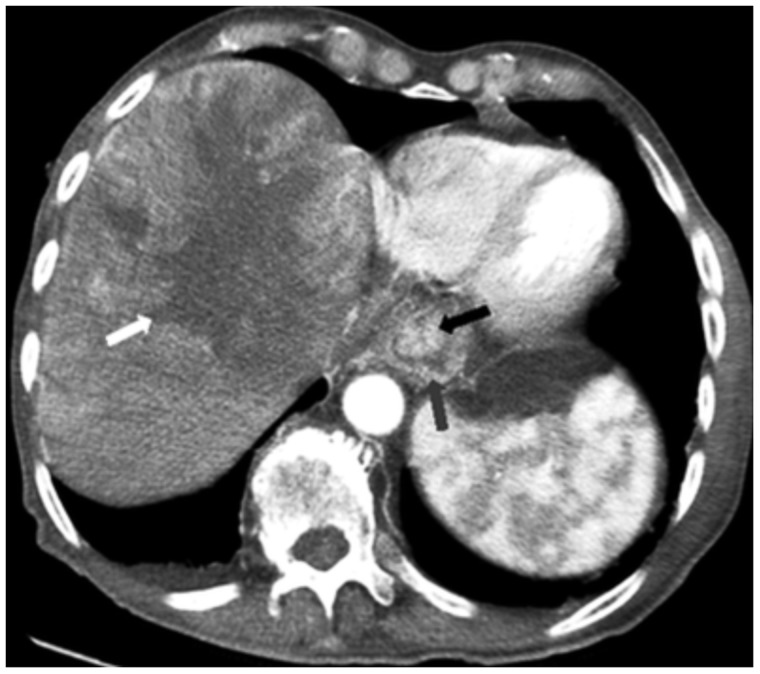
Computed tomography scan reveals a 12.6 × 11.2 cm liver mass with central necrosis and heterogeneous enhancement (white arrow) and esophageal wall thickening (gray arrow) with a large luminal mass (black arrow) almost obstructing the lumen.
